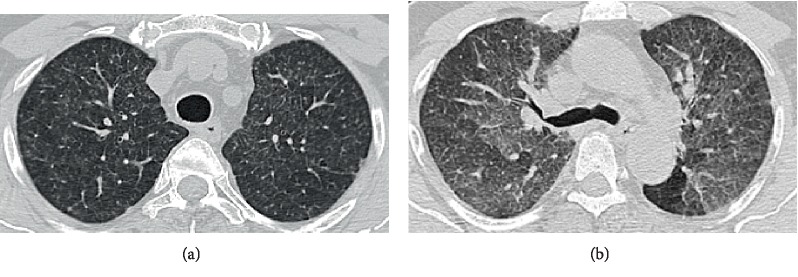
HRCT demonstrating upper lobe predominant centrilobular nodules of ground glass density (a). Expiratory HRCT slices show air trapping in lobules that had decreased attenuation on inspiratory slices with centrilobular nodules of ground glass density (b).
